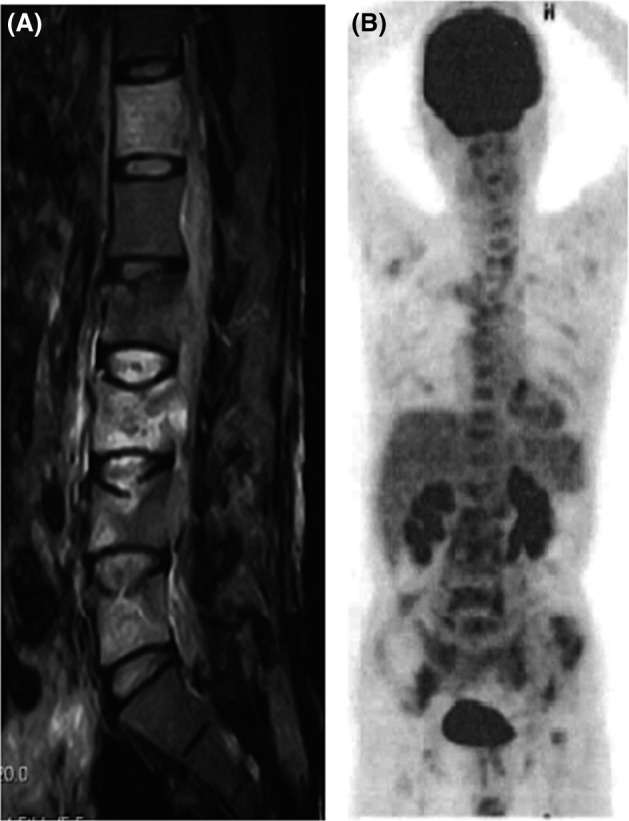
A, Sagittal T2‐weighted magnetic resonance imaging of the spine. A tumor emerging from the vertebral bodies of L2 through L4 is present. In addition, high signal intensity in the vertebral bodies of Th12, L3‐L5 is evident. B, 18F‐fluoro‐deoxy‐glucose positron‐emission tomography image showing nodular uptakes in the vertebras, ribs, pelvis, and femur. Standardized uptake value given as the maximum pixel value in the tumor was 3.54 at the right pelvis, where it was increased the most
